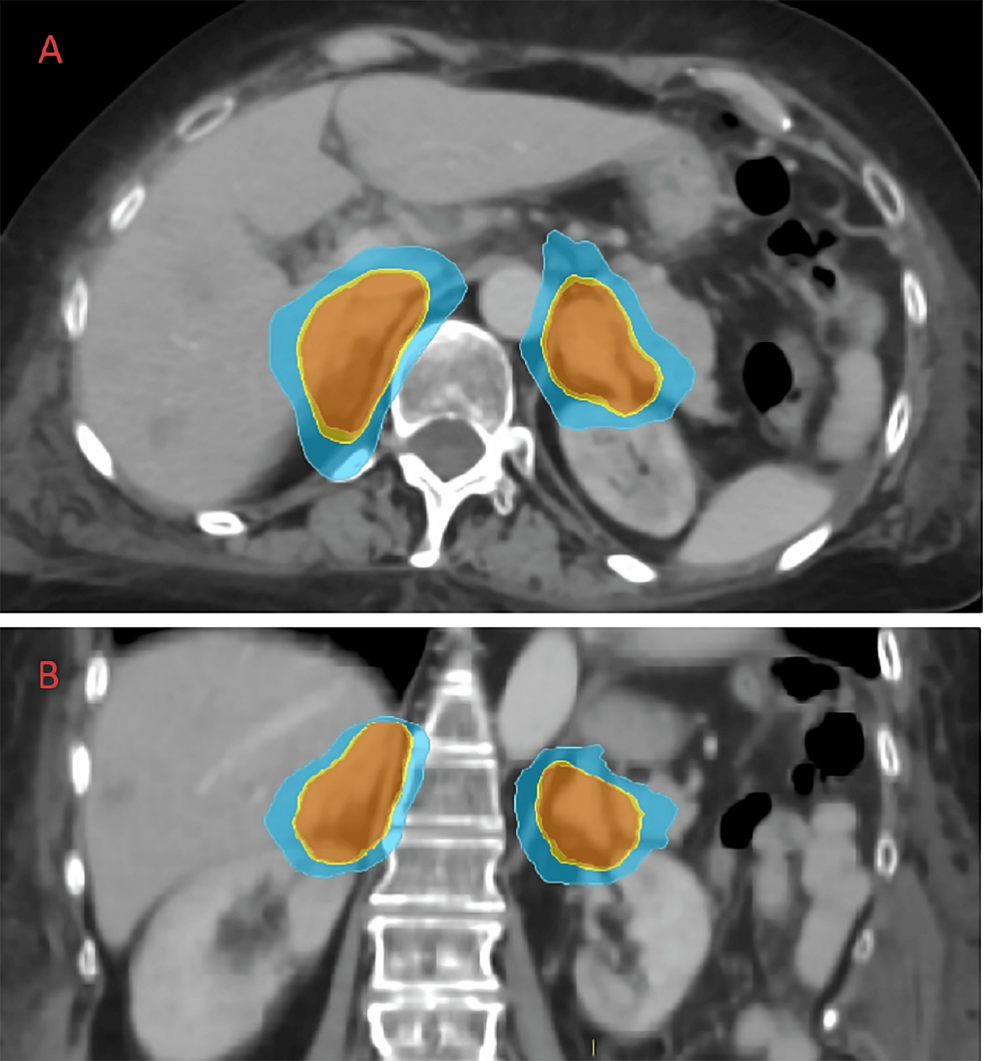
Radiotherapy treatment planning CT axial plane image (A) and coronal plane image (B) showing isodose levels overlying the adrenal glands: 3,900 cGy/100% (orange), 3,705 cGy/95% (yellow), 3,000 cGy/77% (green), and 2,730 cGy/70% (blue)
